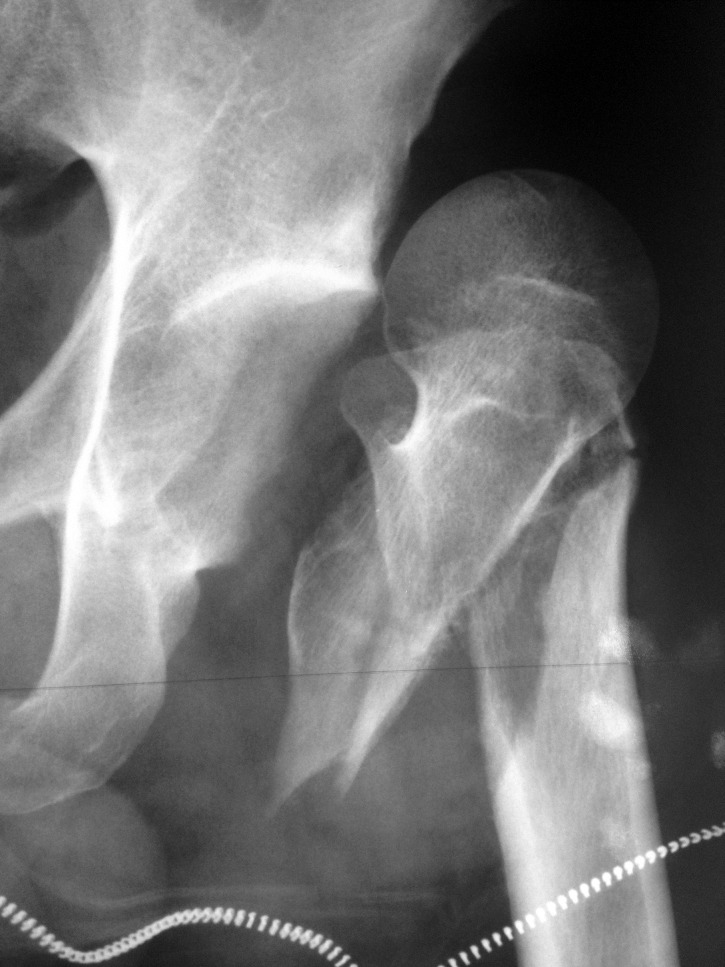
Antero-posterior X-Ray view of the left hip showing anterior displacement of the femoral head and intertrochanteric fracture
